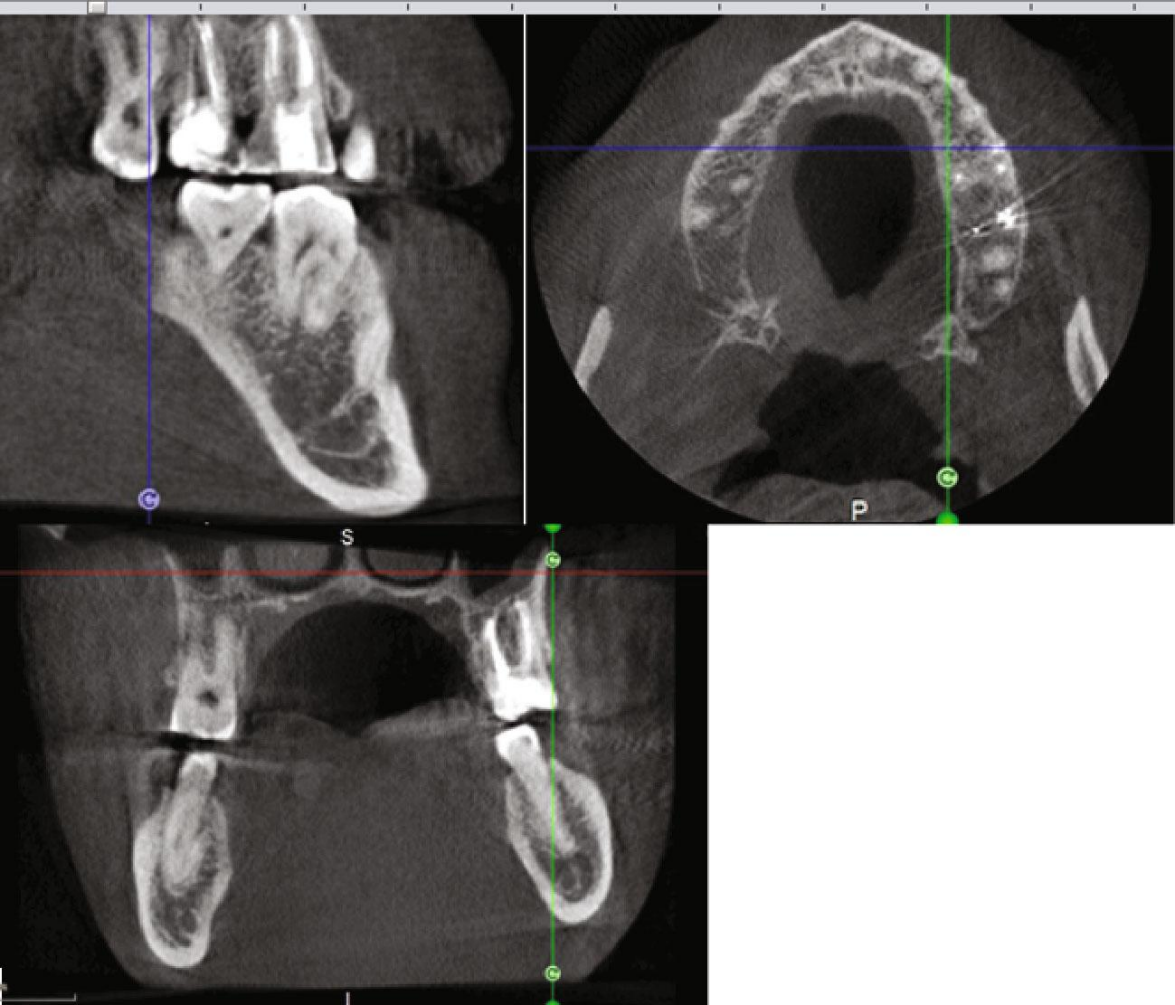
CBCT after 10 months of obturation.
